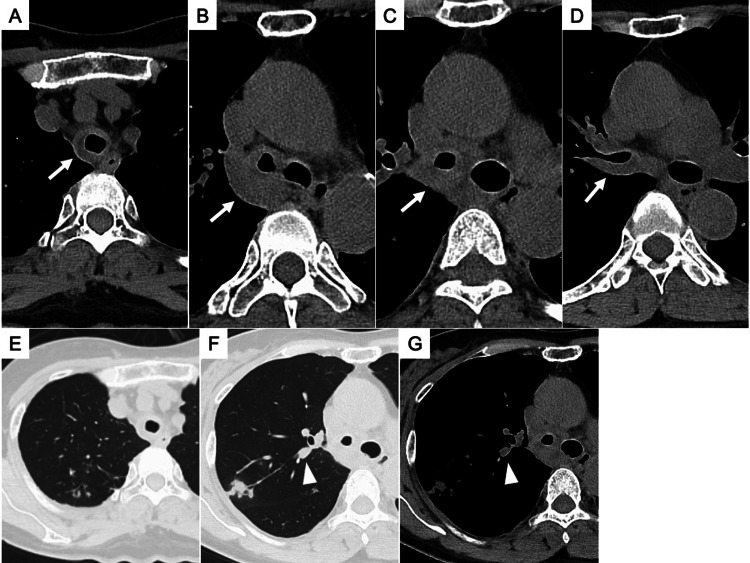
Chest computed tomography findings at the initial presentation. Chest computed tomography at the initial presentation shows circumferential wall thickening (white arrow) in the trachea (A), carina (B), right main bronchus (C), and right upper lobe bronchus (D). Granular and nodular shadows in the right S^2^ of the lung (E, F), and a nodular shadow that appears to be mucoid impaction in the orifice of the right B^2^ (white arrowhead) are also seen (F, G).
